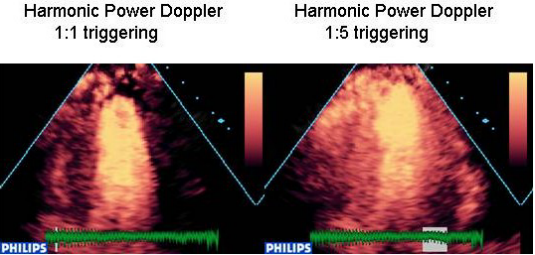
Harmonic power Doppler imaging [see also additional file 1]
